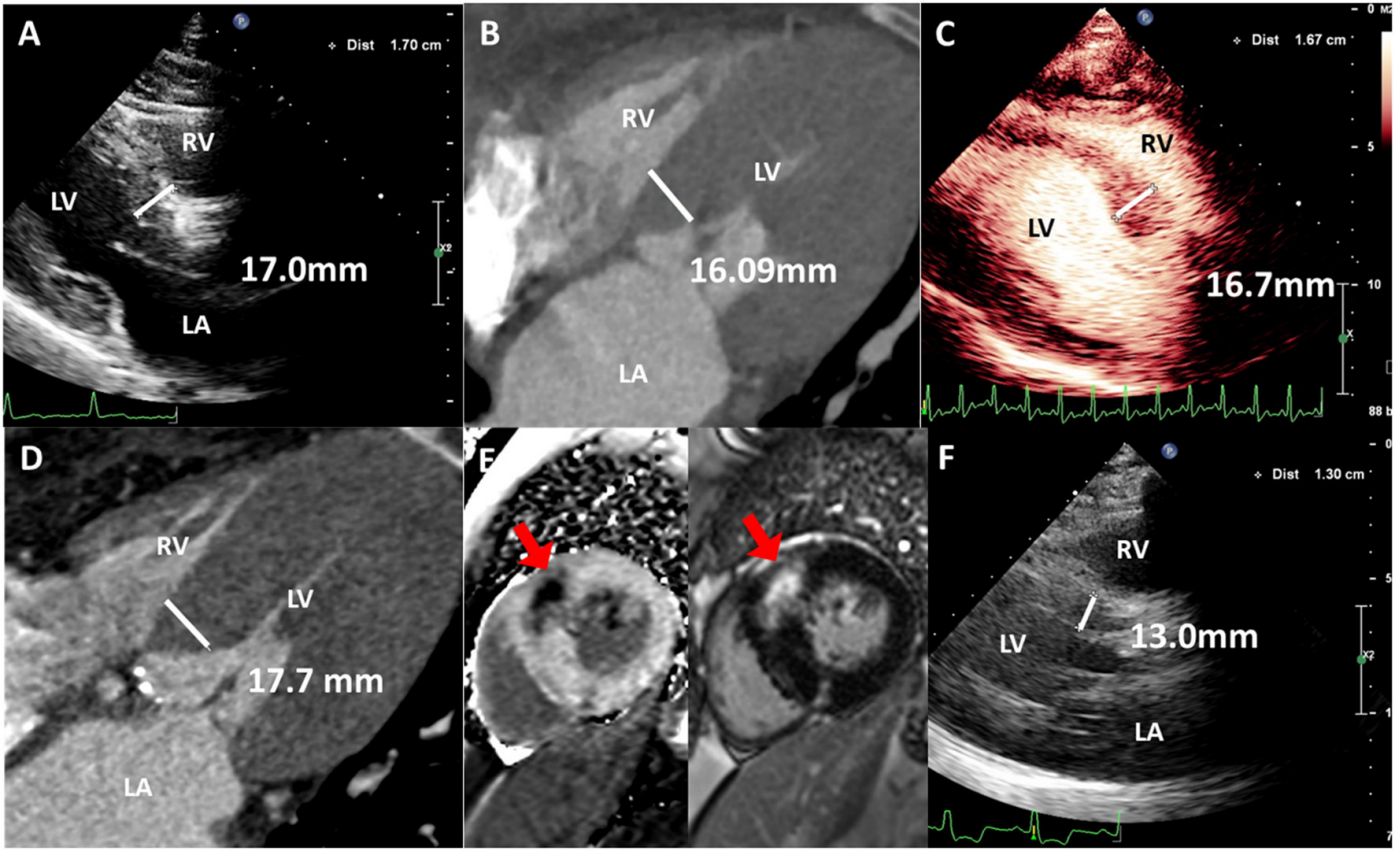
Imaging characteristics of interventricular septa. (A) Thickened interventricular septa was confirmed by TTE of 17.0 mm. (B) Thickened interventricular septa was confirmed by CT of 16.09 mm. (C) Left ventricular contrast echocardiography shows thickened interventricular septa of 16.7 mm at 6-month follow-up after TAVR. (D) Thickened interventricular septa was confirmed by CT of 17.7 mm at 6-month follow-up after TAVR. (E) Myocardial fibrosis and delayed enhancement was confirmed by cardiac magnetic resonance. (F) Interventricular septal thickness decreased to 13.0 mm after PIMSRA 5 days later. Red arrows: delayed enhancement.
